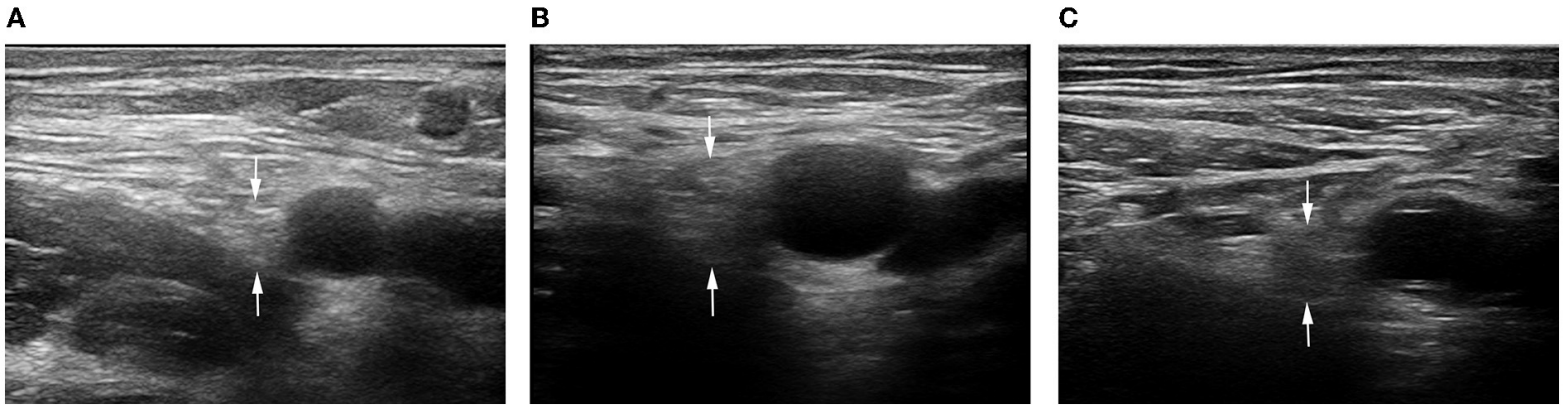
2D ultrasound image of the FN. (A) Control subject; (B) patient with T2DM, non-DPN group; (C) patient with T2DM, DPN group.
